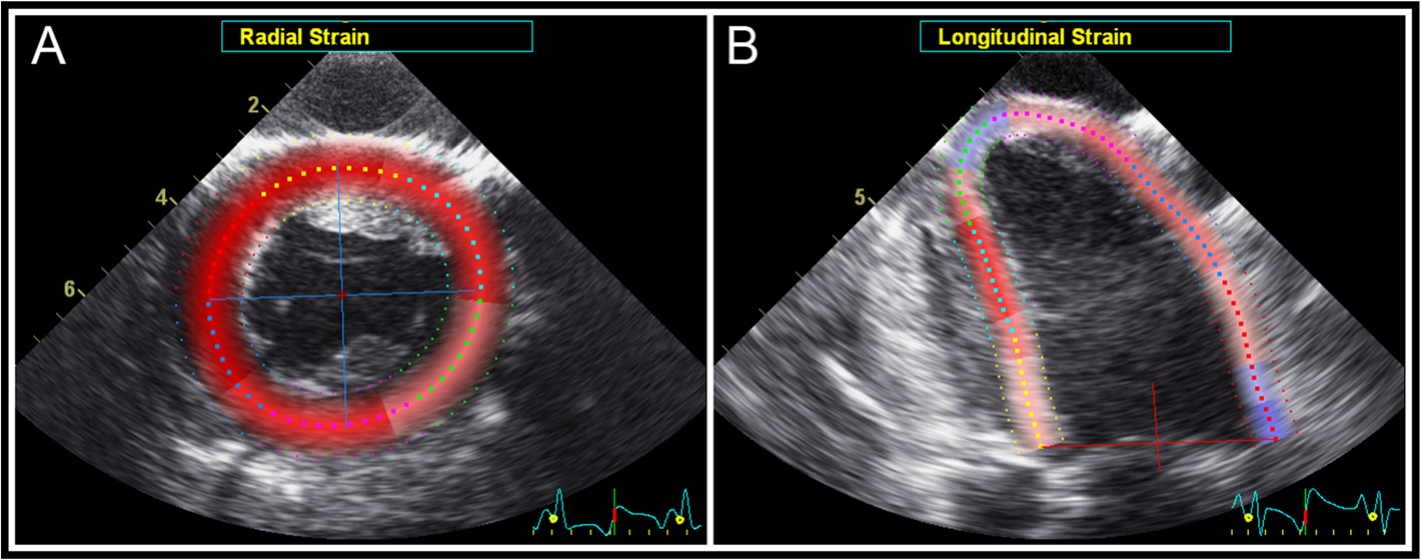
Speckle Tracking Echocardiography (STE). (A) Epicardial left ventricular short-axis view used for STE. During analysis, knots (square-like points) are evenly distributed along the middle of the region of interest and the region of interest is itself divided into six standardized segments. Tracking of these knots from frame to frame is used for estimation of radial and circumferential strain in each segment. The anterior and posterior wall was defined as the yellow and pink segment, respectively. (B) Epicardial left ventricular long-axis view used for STE. The same principle is used for estimation of longitudinal strain in each segment. The anterior and posterior wall was defined as the dark blue and cyan segment, respectively.
